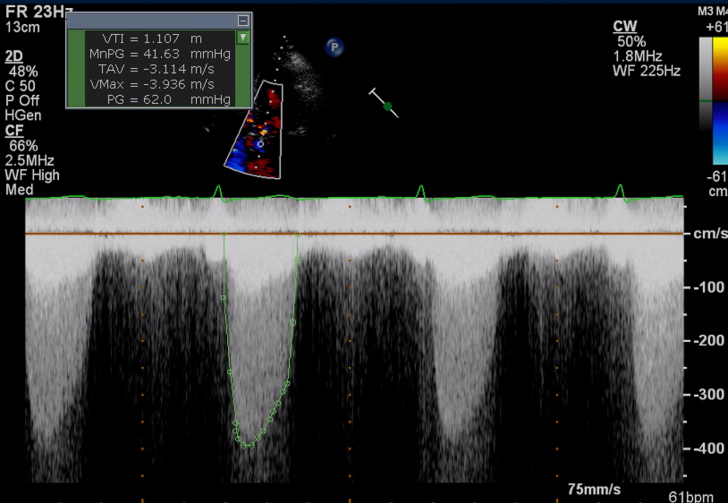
Aortic Velocities Using Ultrasound Enhancing Agents CW Doppler evaluation of a patient with aortic stenosis. The gain settings were reduced to be ensure that gradients are not overestimated.5.Recognize LF-LG AS. This occurs when left ventricular stroke volume is low, leading to peak AV velocity or AV gradients that may not fulfill all the criteria for high gradient severe AS. For patients with severe LF-LG AS, the calculated AV area is <1.0 cm^2^, indexed stroke volume is <35 mL/m^2^, and dimensionless index is <0.25. Accurate measurement of the calculated stroke volume in these patients is vital with caution not to overestimate or underestimate the LVOT diameter or the LVOT PW velocities. Dobutamine stress echocardiogram may be ordered to confirm the diagnosis of severe LF-LG AS in certain patients.6.Limitations of the continuity equation include the assumption that the LVOT area is perfectly round in its shape. In settings where the LVOT is elliptical in shape, the measured LVOT diameter in the PSLX view may underestimate the true LVOT area using the continuity equation. In situations where the LVOT is elliptical or eccentric in shape, using a 3-dimensional volume set to measure a direct planimetry area of the LVOT may be a reasonable work around.
